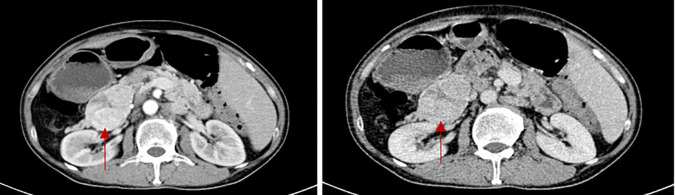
The CT scan of glucagonoma (The red arrow shows the location of the tumor. The two images show the arterial phase of the enhanced CT scan and the portal phase of the enhanced CT scan.)
